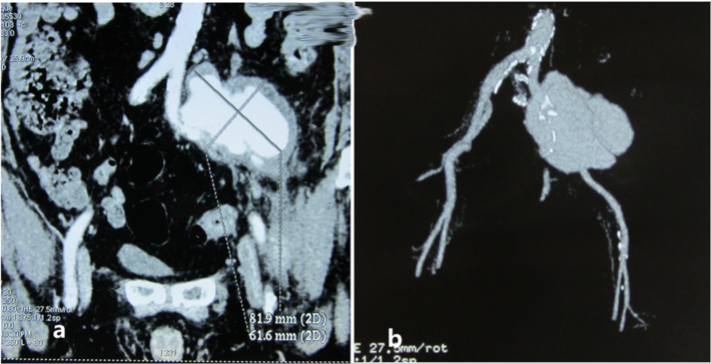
Imaging of computed tomographic angiography before the endovascular procedure. (a) and (b) The contrast-enhanced computed tomographic angiography showed an 81.9×61.6mm lobular hematoma covering the bifurcation of the left common iliac artery and the proximal ends of both internal and external iliac arteries.
